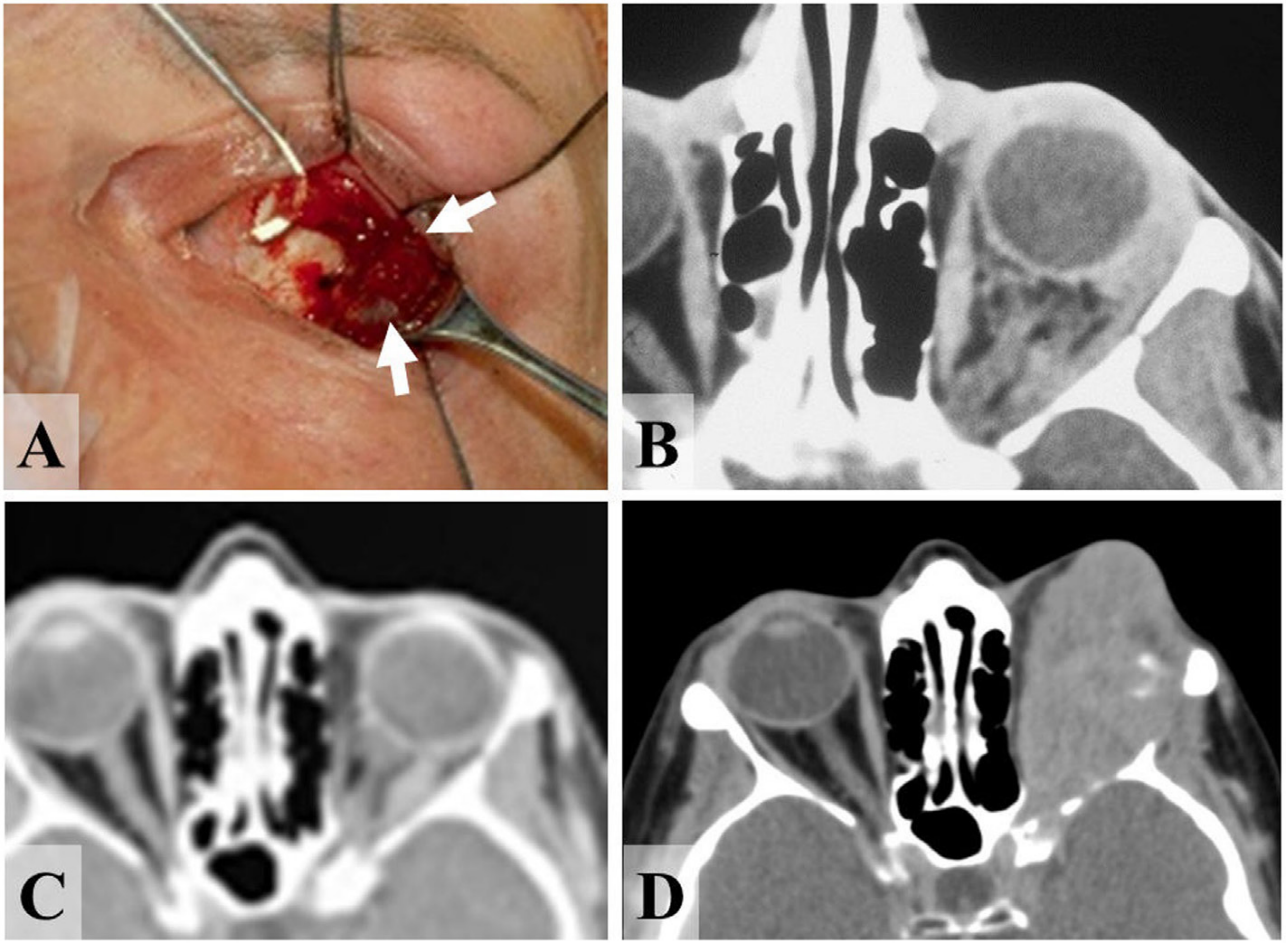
47-year-old female presenting with primary malignant melanoma of the left orbit. (A) Melanoma (arrows) throughout orbital fat alongside the lateral rectus (on squint-hook) at time of incisional biopsy, and (B) CT scan of orbits prior to diagnostic biopsy, showing diffuse tumor infiltration of the left retrobulbar fat. (C) CT at 8 years after orbital radiotherapy, showing the inactive orbital disease that persisted for more than 13 years. (D) After 13 years there was a very rapid recurrence of tumor, from which the patient died about a year later.
